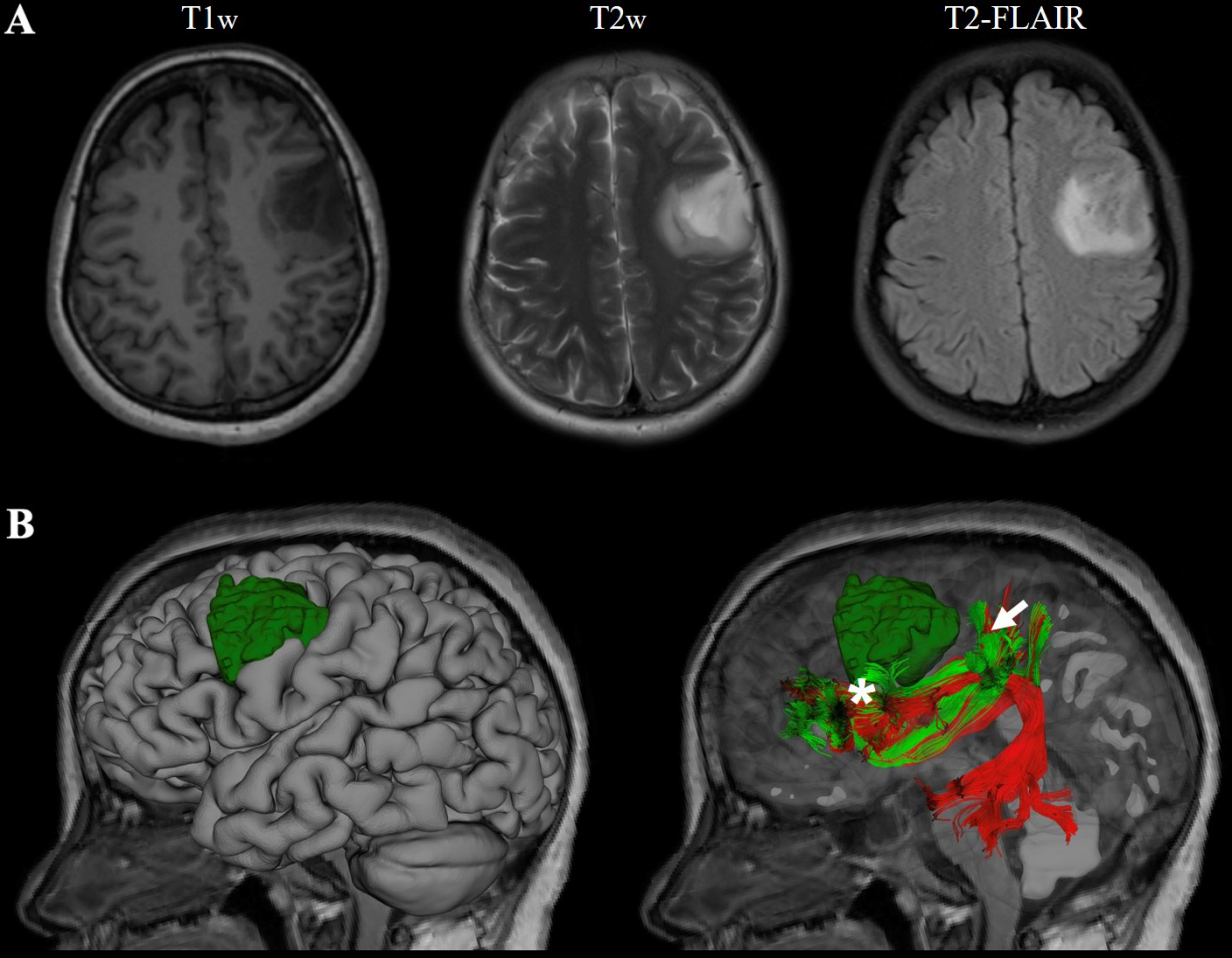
Representive imaging of a patient with left frontal lobe astrocytoma. Case 3, a 41-year-old female with left frontal lobe astrocytoma (WHO grade III) in MR images (A). The relationship between language-related fiber tracts (AF in red and SLF-II in green color) and tumor (dark green) were shown in (B); the eloquent area of intro-operative speech arrest (DCS) was marked with a star(Projecting terminal territory of AF) and an arrow (Projecting terminal territory of SLF-II).
